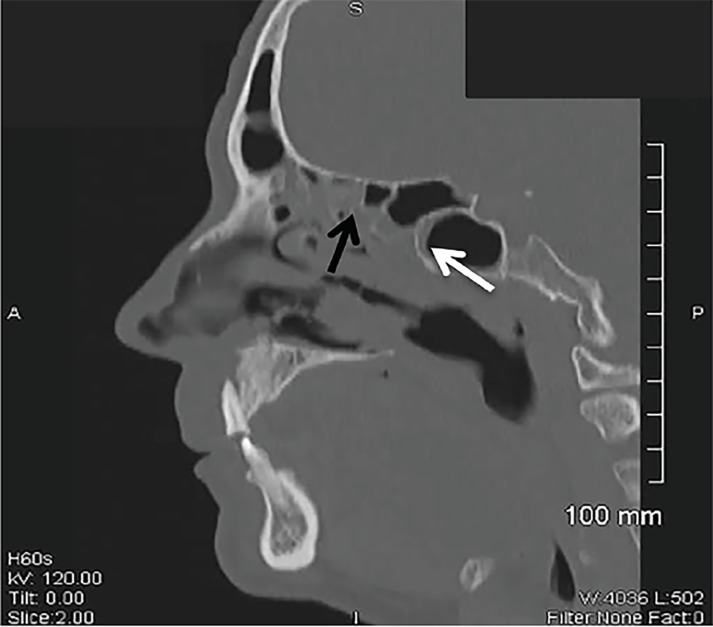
Sagittal view of a computed tomography scan of the facial bones revealed extensive acute and chronic sinusitis of the sphenoid (white arrow) and ethmoid sinuses (black arrow).
